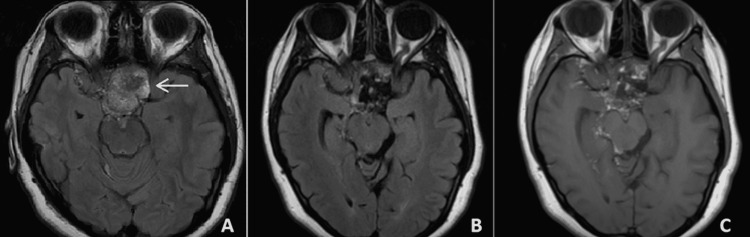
MRI axial views of the dermoid cyst (straight arrow) on preoperative FLAIR (A), postoperative FLAIR (B), and non-contrast T1 (C) sequences.
